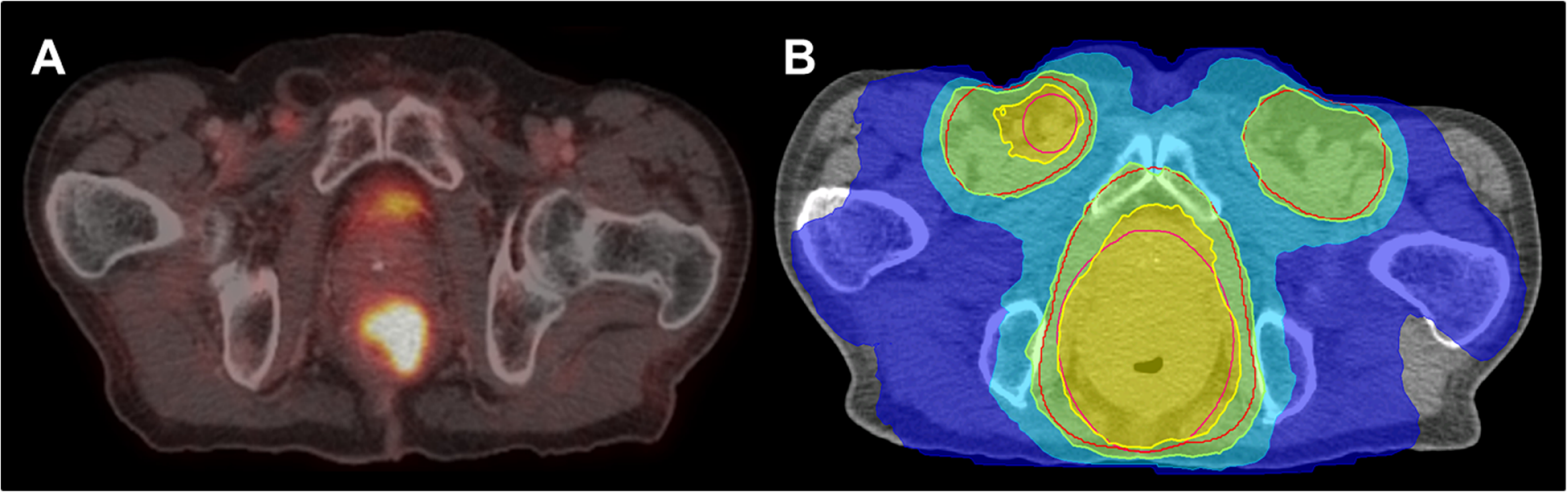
A 68-years old male patient with newly diagnosed anal cancer Stage IIIC (cT4 cN1 cM0) and ^18^F-FDG PET/CT with ^18^F-FDG avid primary tumor and inguinal lymph node (a). ^18^F-FDG PET/CT was then used for radiotherapy planning with boost to the right inguinal lymph nodes and primary tumor (b)
